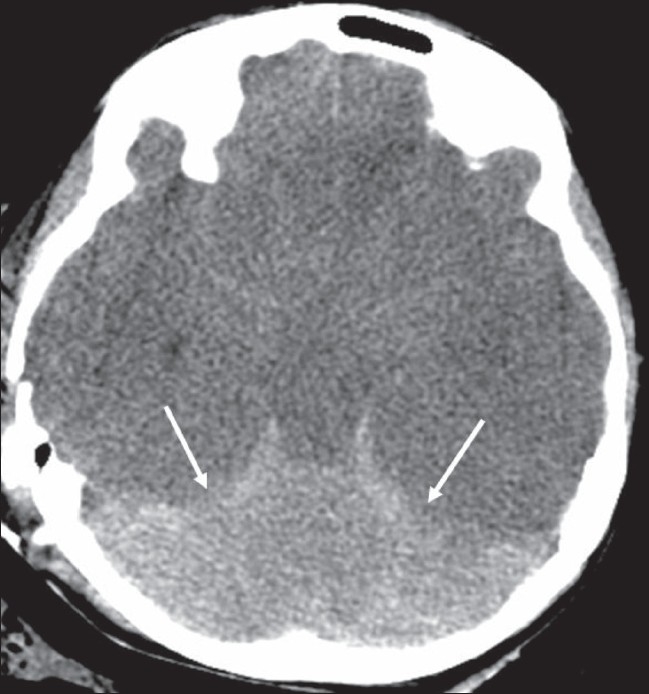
Reversal sign. Axial noncontrast CT scan of the brain in a child with severe head injury shows low attenuation of the cerebral hemispheres with complete loss of gray matter-white matter differentiation, small temporal horns, and effacement of the basal cisterns. The findings are suggestive of cerebral edema. There is relative preservation of the cerebellum (arrows) with hyperattenuation (‘white cerebellum’)
